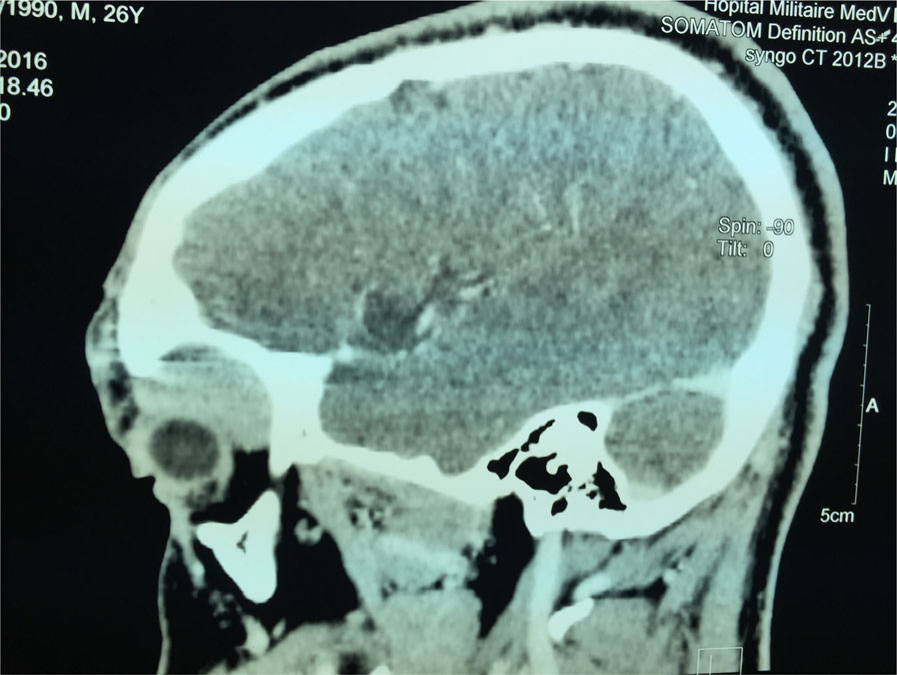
Computed Tomography scan showing a well circumscribed and homogeneous left supraconal tumor
